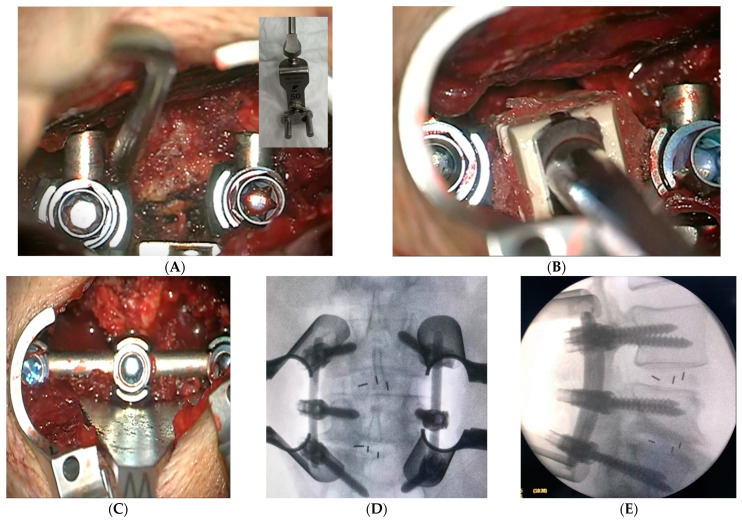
(A) Special retractor is applied to distract the two adjacent pedicle screws’ heads. (B) Following a sufficient decompression, the cage is then applied. (C) The rods are inserted, and the final tightening is carried out, along with posterolateral decortication and bone graft application. (D,E) AP and lateral radiographs are taken to confirm the pedicle screws and cage position.
